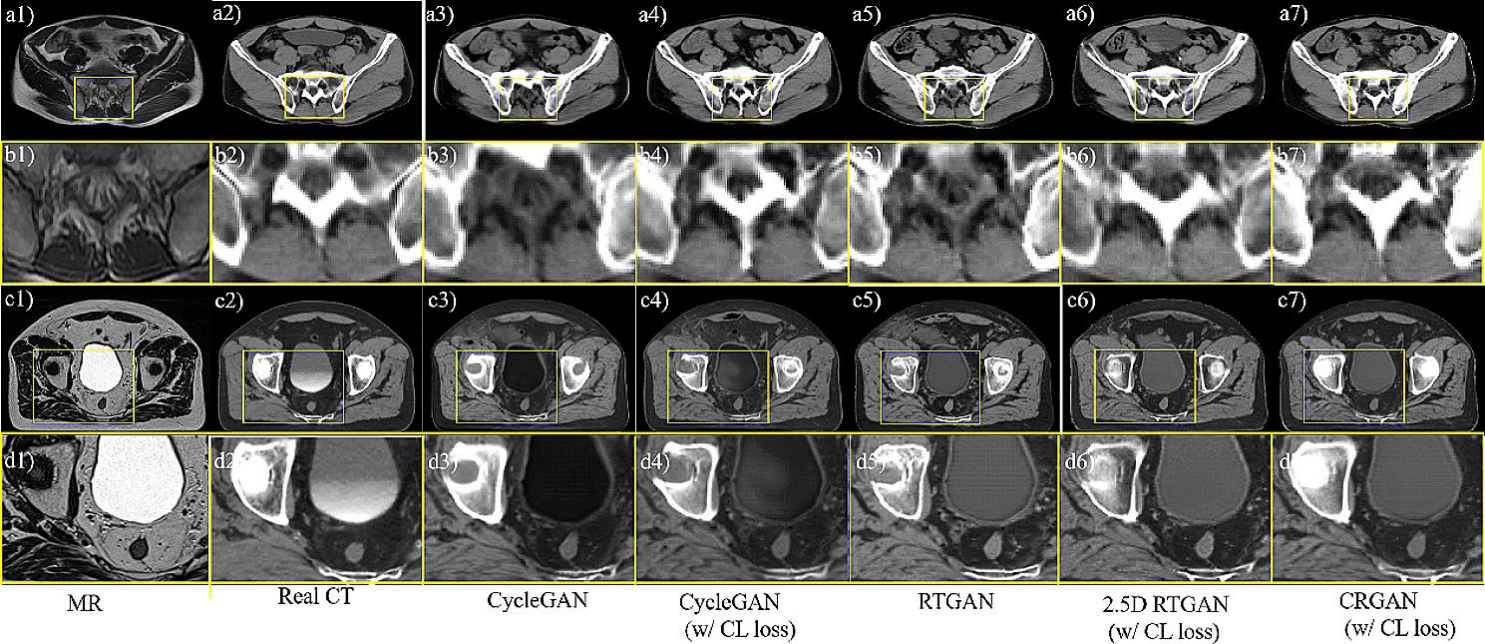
sCT images generated by different model. The first and third rows show real MR (a1, c1), real CT (a2, c2) and the sCT images generated by CycleGAN (a3, c3), CycleGAN with CL loss (a4, c4), RTGAN (a5, c5), 2.5D RTGAN with CL loss (a6, c6) and our model (a7, c7). The second and fourth rows highlight the ROIs outlined by the yellow box on each corresponding image
